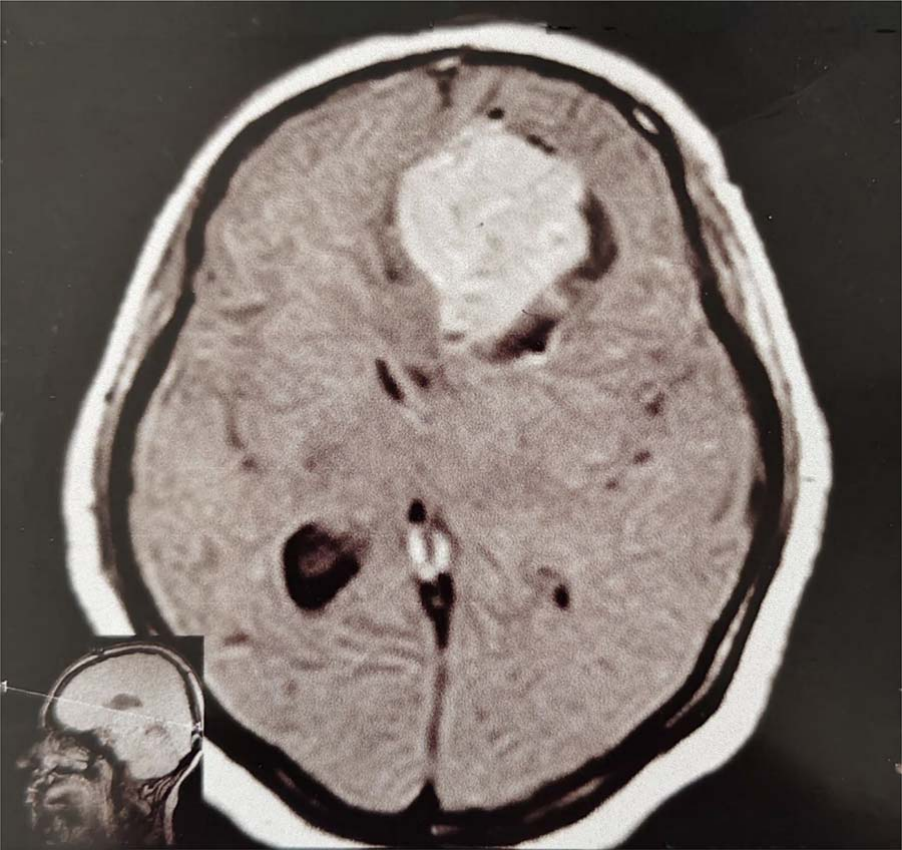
MRI brain without IV contrast (T1 sequence) showing a well-circumscribed hypointense mass with areas of heterogeneity occupying the right frontal lobe, closely related to the midline structures and falx cerebri.
